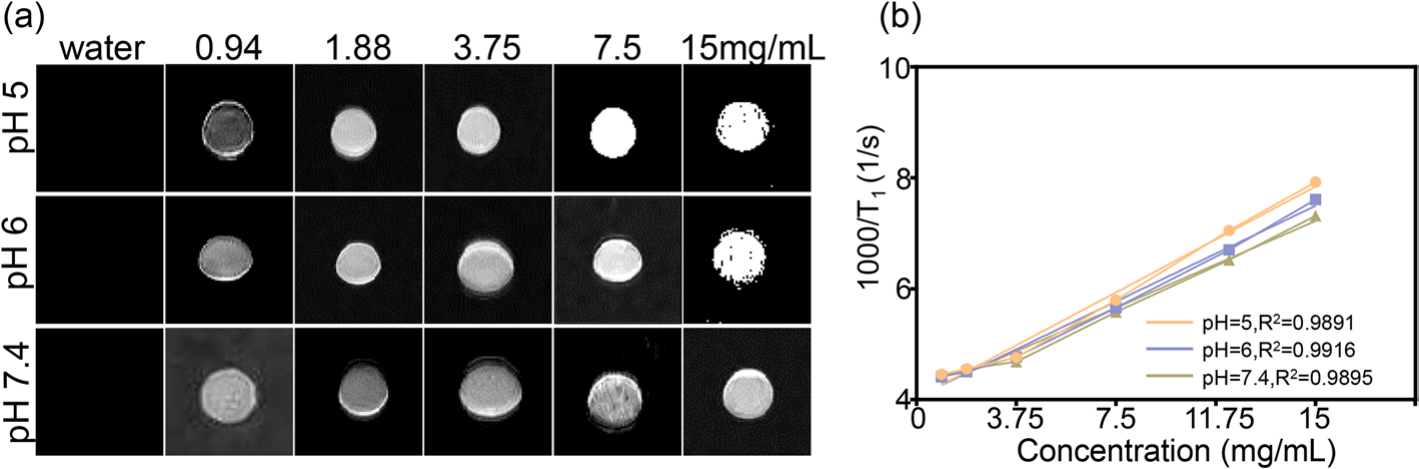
In vitro MRI of MHCF NPs. a MRI of MHCF NPs at different concentrations and pH values. b MRI relaxation rates of MHCF NPs at different concentrations and pH values
